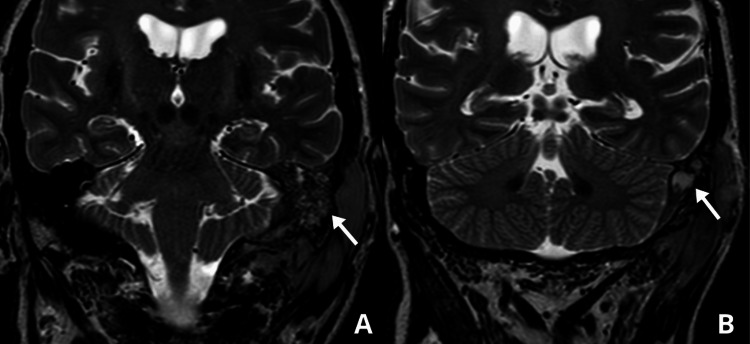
Pre-biopsy brain MRI (coronal view) Heterogeneous lesion (2 x 1.3 cm) occupying the left external auditory canal with extension to the retroauricular region, surrounding the mastoid (arrow in panel A), and involving the facial nerve at the stylomastoid foramen (arrow in panel B).
